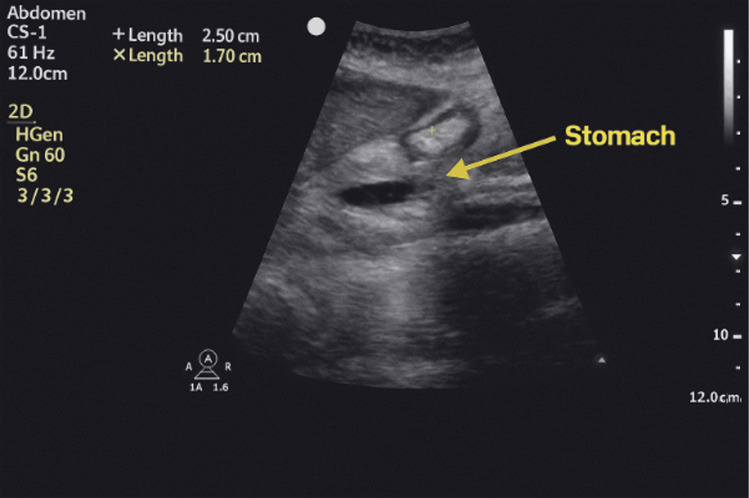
Preoperative gastric ultrasound Arrow indicates the stomach, demonstrating absence of gastric contents.
